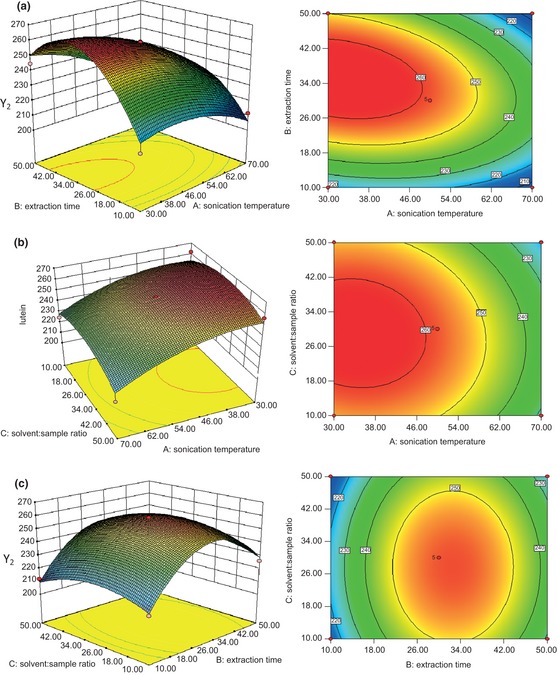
RSM contour and 3D surface plots of the effects of UAE conditions on lutein concentration. (a) Solvent:sample ratio (30:1); (b) time (30 min); (c) temperature (50°C). RSM, response surface methodology; 3D, three‐dimensional; UAE, ultrasonic‐assisted extraction.
